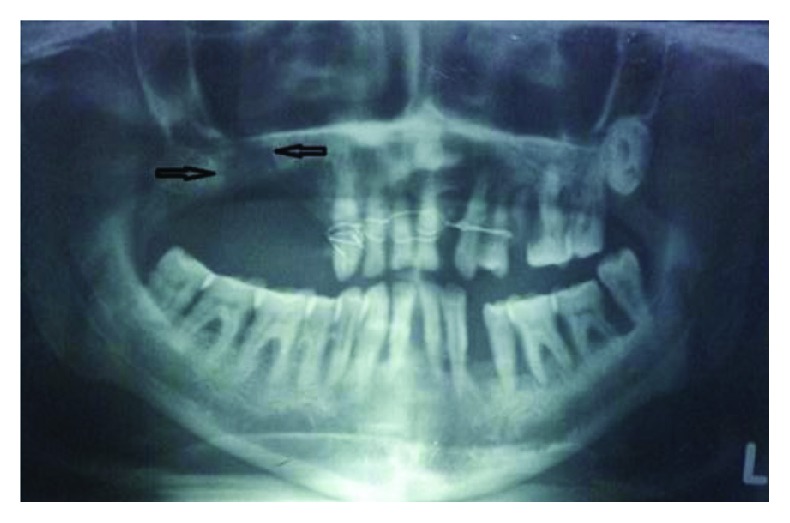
Panoramic X-ray demonstrating the underlying osseous defect.
